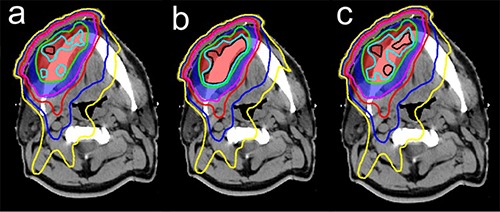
Dose distributions calculated for patient #1 using CT‐derived density (a), homogeneous density (b), and bulk density (c). The red shaded region is primary PTV and the blue is secondary PTV. Primary PTV was prescribed at 7000 cGy, secondary PTV was prescribed at 5600 cGy. The isodose lines shown are 7490 cGy (black), 7350 cGy (light blue), 7000 cGy (green), 5600 cGy (purple), 4900 cGy (red), 3500 cGy (blue), and 2500 cGy (yellow).
